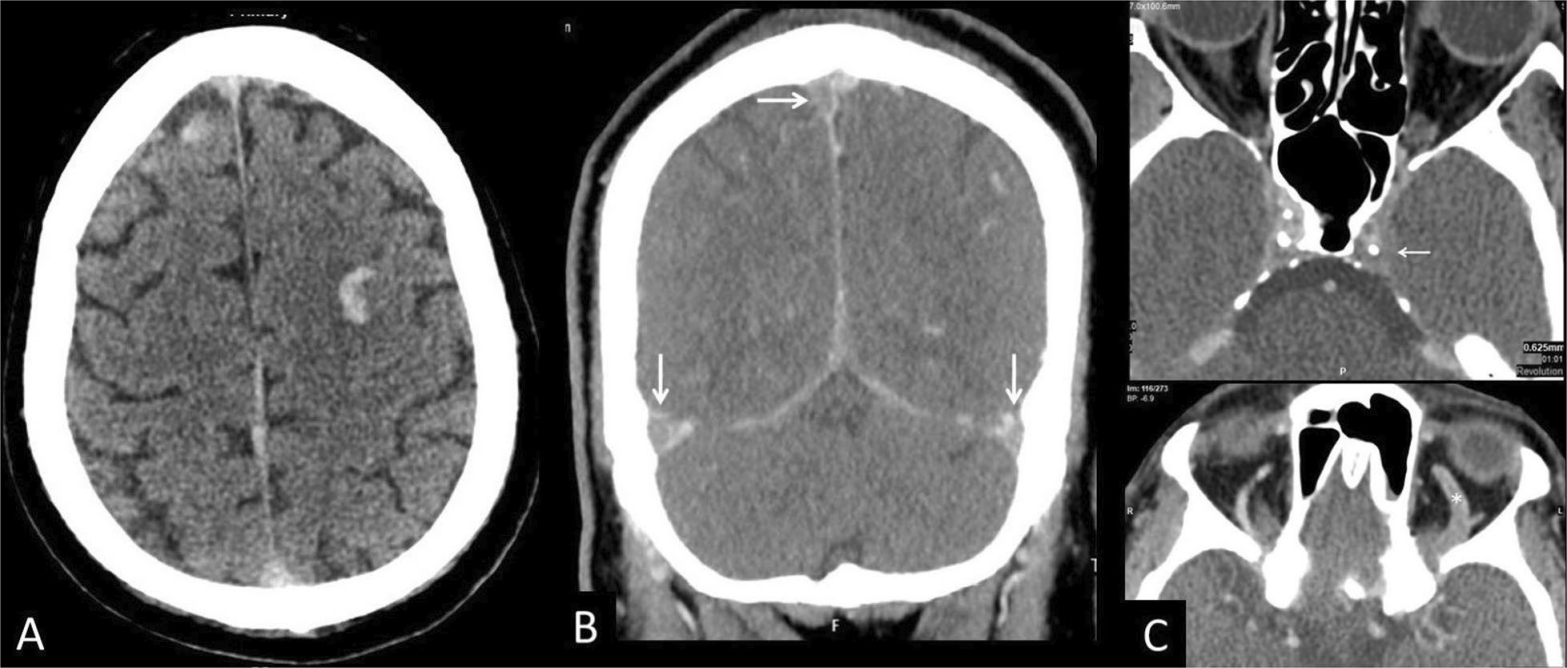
Venous sinus thrombosis. 60-year-old man presented with acute confusion. a Axial non-contrast CT revealed bilateral frontal hemorrhagic infarctions. b CTV demonstrating dural sinus thrombosis involving the superior sagittal sinus, bilateral transverse, and sigmoid sinuses (arrows). c The left cavernous sinus is also thrombosed (white arrow) with extension to the left superior ophthalmic vein (asterisk)
